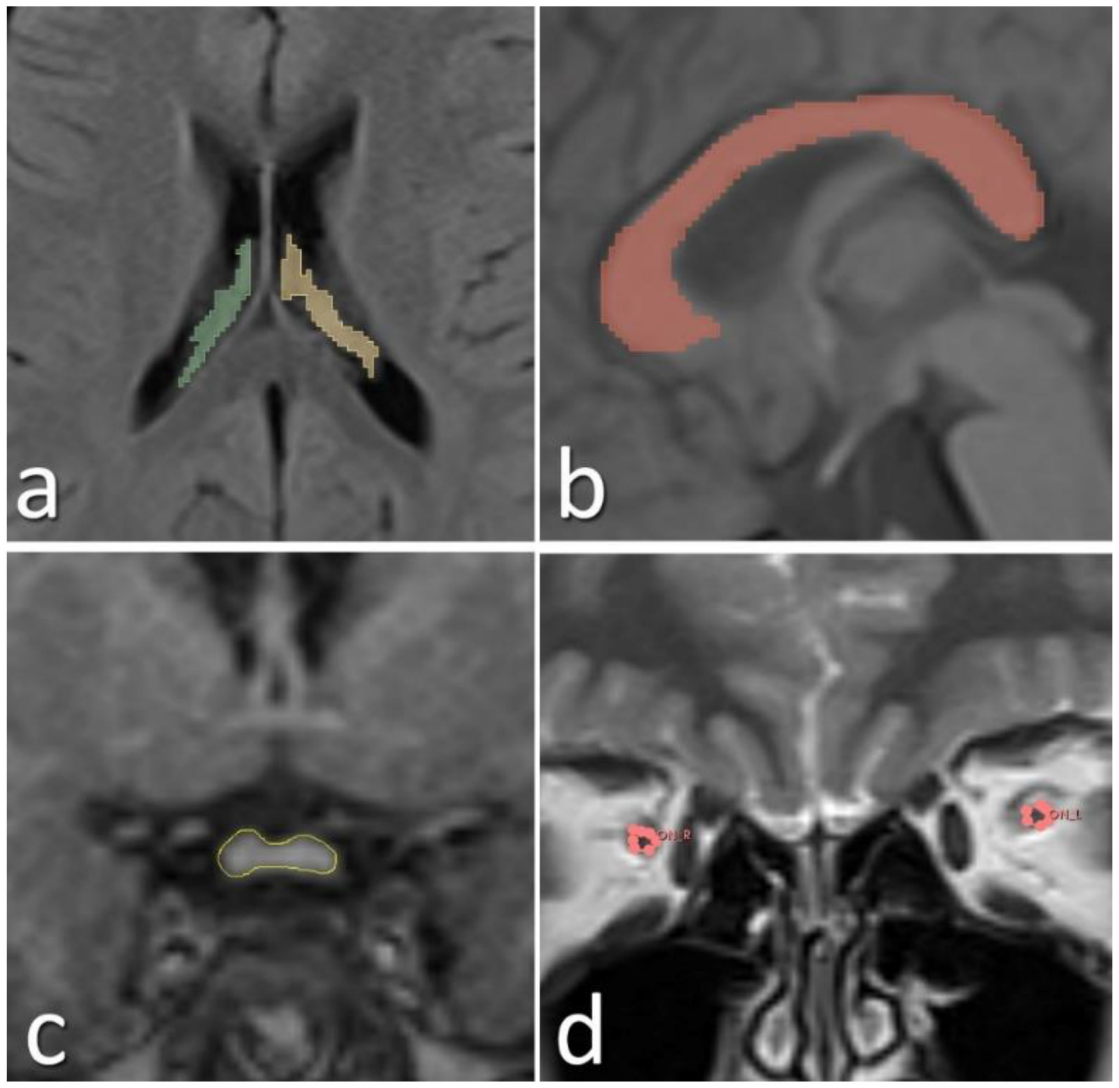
Manually segmented choroid plexus (a), corpus callosum (b), optic chiasm (c), and optic nerves (d) from the acquired MR images are shown.
